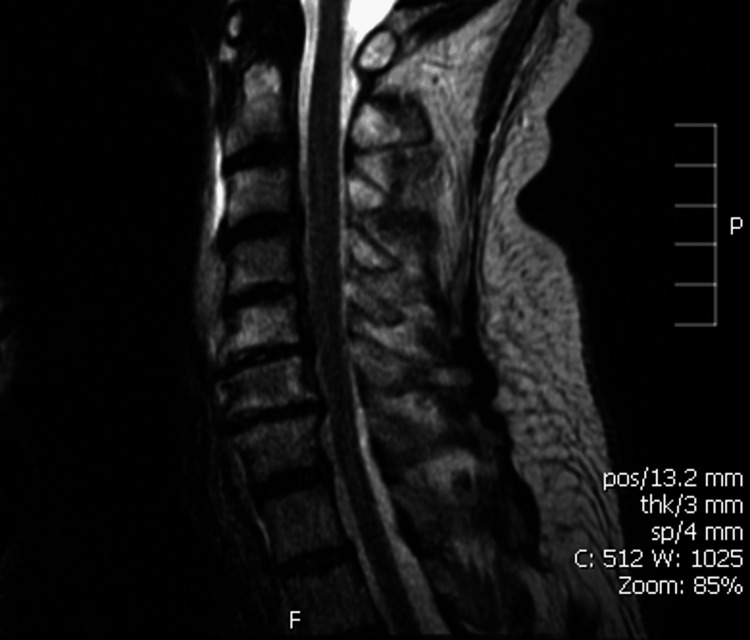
Case D: MRI before surgery. There is moderate to marked right C6 root compression. There is mild posterior osteophyte formation at C6-7 causing mild thecal sac deformity and moderate asymmetric left C7 root compression.
